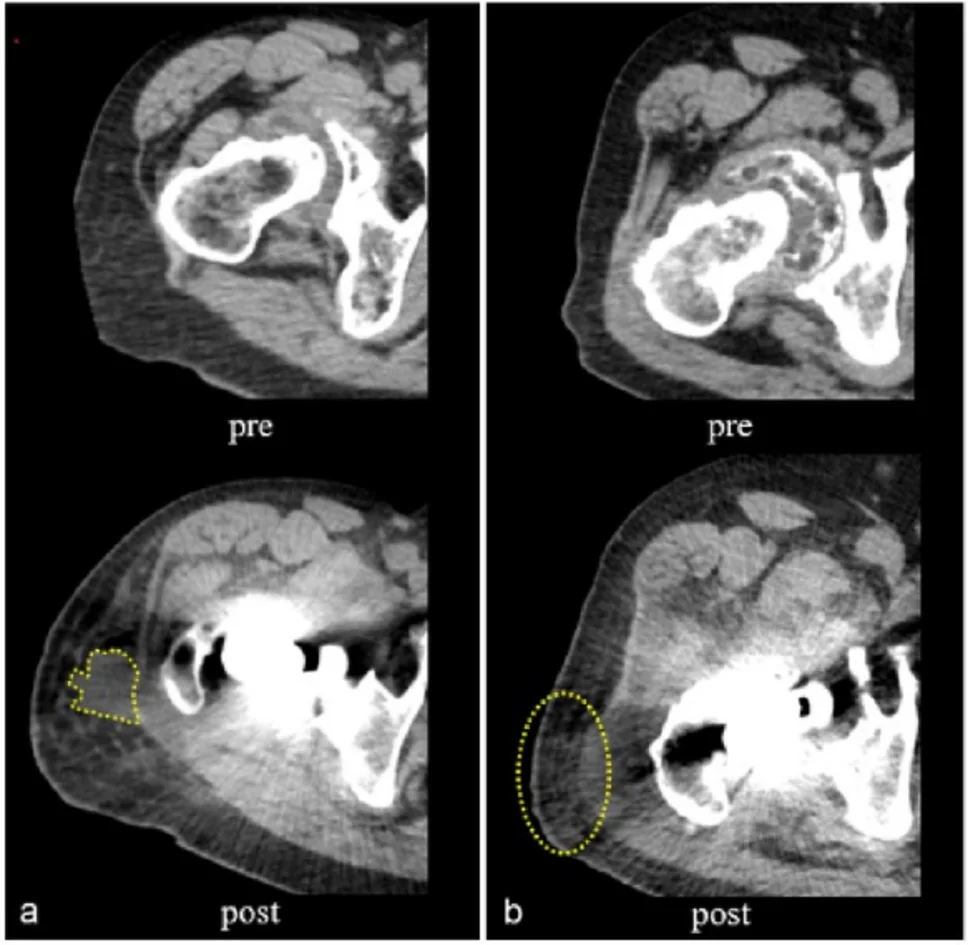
Two representative cases with and without subcutaneous hematoma. (a) Case with subcutaneous hematoma, outlined by a yellow dashed line. (b) Case without subcutaneous hematoma; the expected location is outlined by a yellow dashed line. Preoperative and postoperative computed tomography scans are shown
